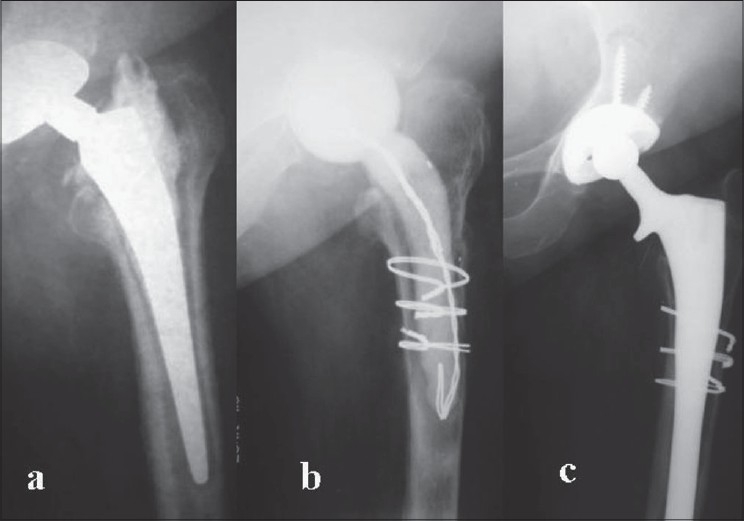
Two-stage revision of infected bipolar arthroplasty. (a) Preoperative radiograph showing septic loosening of the cemented bipolar prosthesis, (b) First stage of revision surgery with insertion of antibiotic-impregnated cement spacer; fracture of the proximal femur was stabilized with cerclage wiring, (c) Second stage revision surgery with a long hydroxyapatite coated femoral stem
